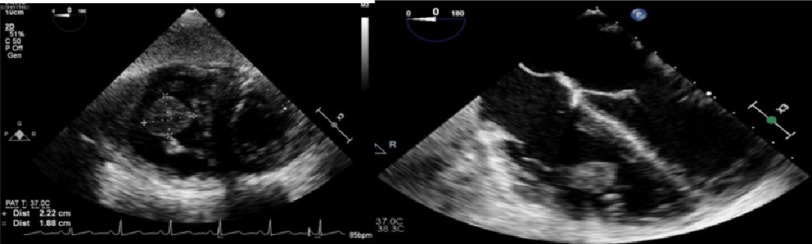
Transesophageal echocardiography shows a 2.2 * 1.9 cm mobile mass likely adherent to sub valvular chordae in the right ventricle.
